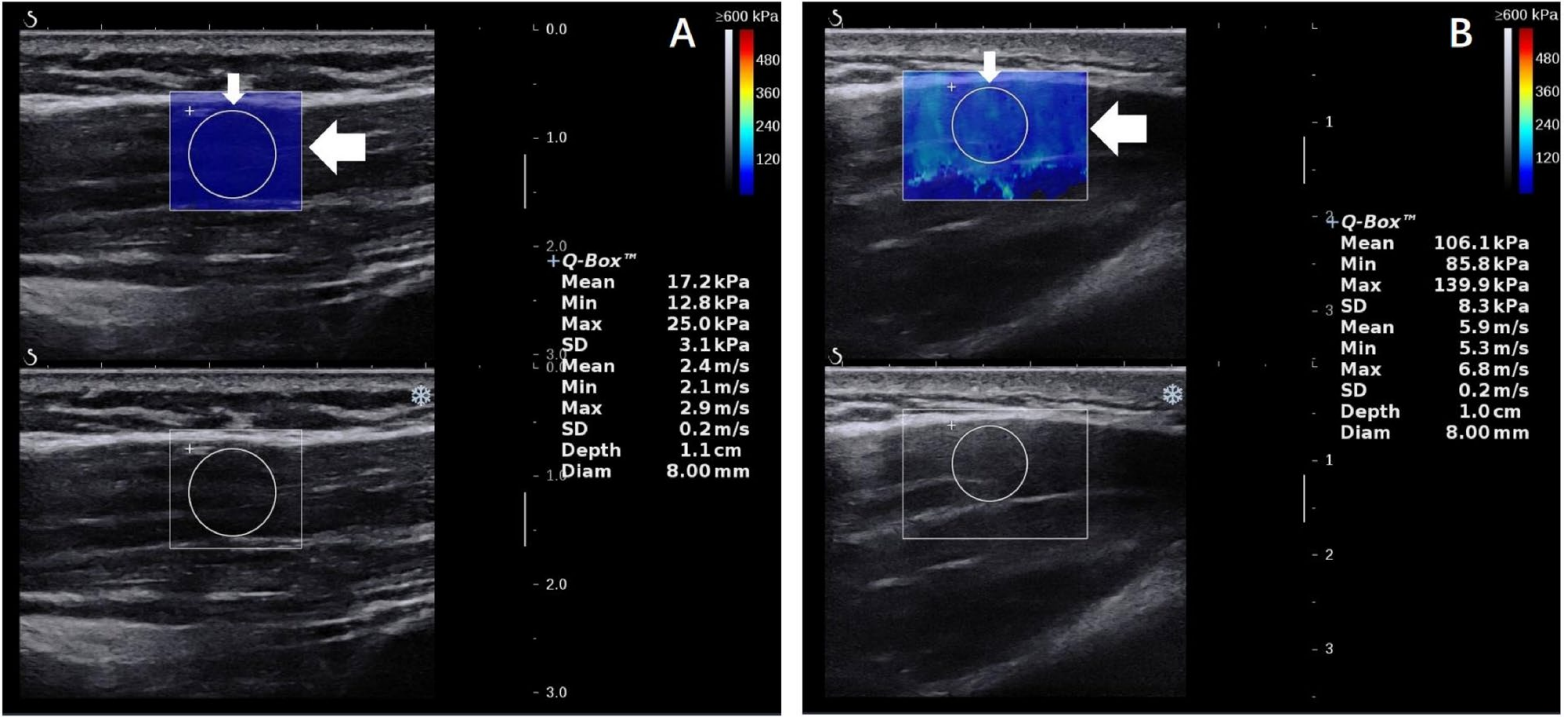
SWE (top panel) ultrasound images of the MD. A color map of MD elasticity is shown in the round Q-box (thin arrow) and square ROI (thick arrow). *MD* middle deltoid, *SWE* shear wave elastography, *SWV* mean shear wave velocity.
